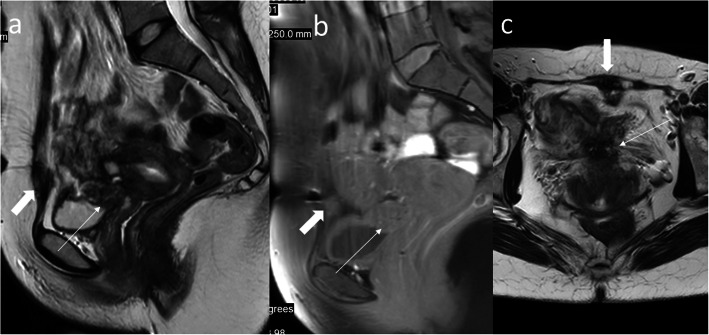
Pelvic MRI consulted for Sports Medicine Centre, Warsaw (images published with permission). FSE/T2WI in the sagittal (a) and axial (c) planes, FSE, T1 fat-saturated image, and the sagittal plane (b). Endometriotic implants on the way of C-section: in the bladder wall (thin arrows) and in the abdominal wall (thick arrows). The lesions are mostly T2-hypointense and T1-isointense, with very tiny foci of hyperintensity
